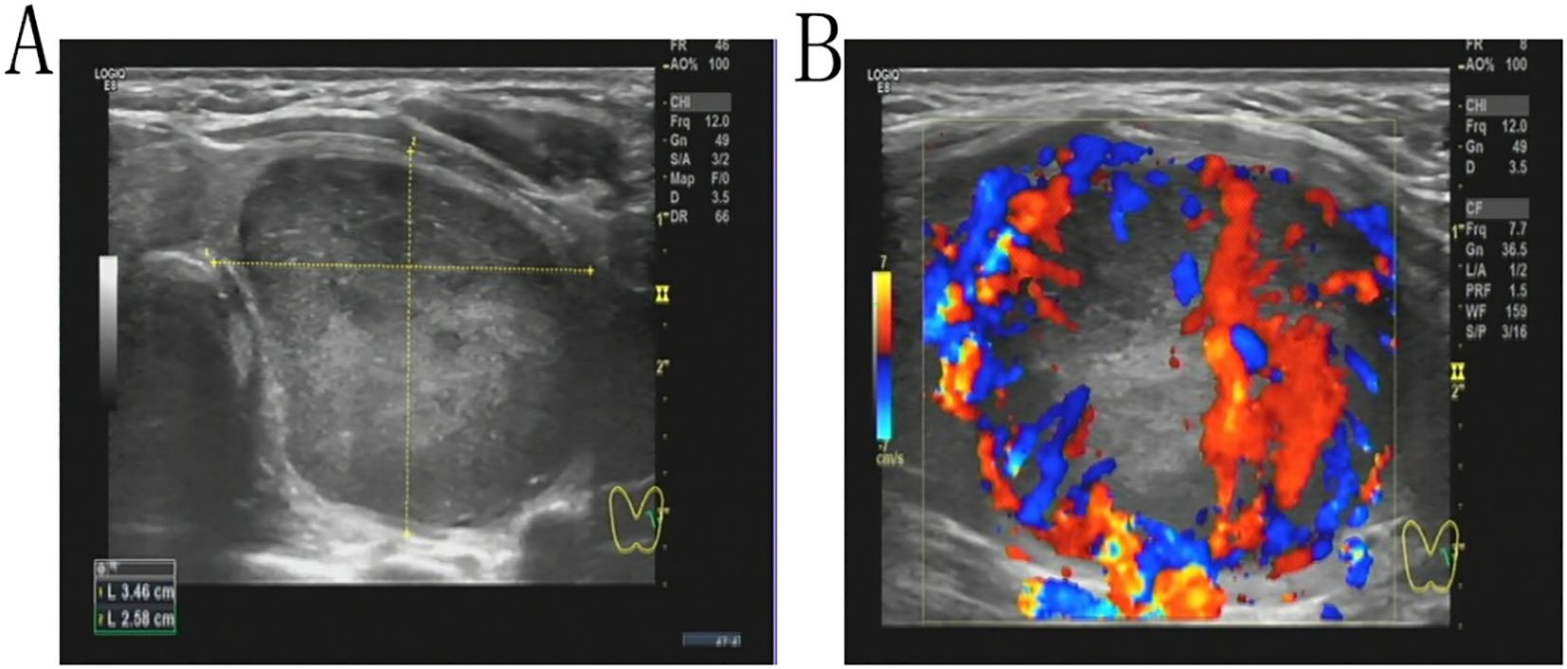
A solid hypoechoic mass measuring 3.46 cm × 2.58 cm × 4.55 cm is present in the midportion of the left thyroid lobe (A). Color Doppler Flow Imaging (CDFI) demonstrates increased vascularity within the mass (B).
